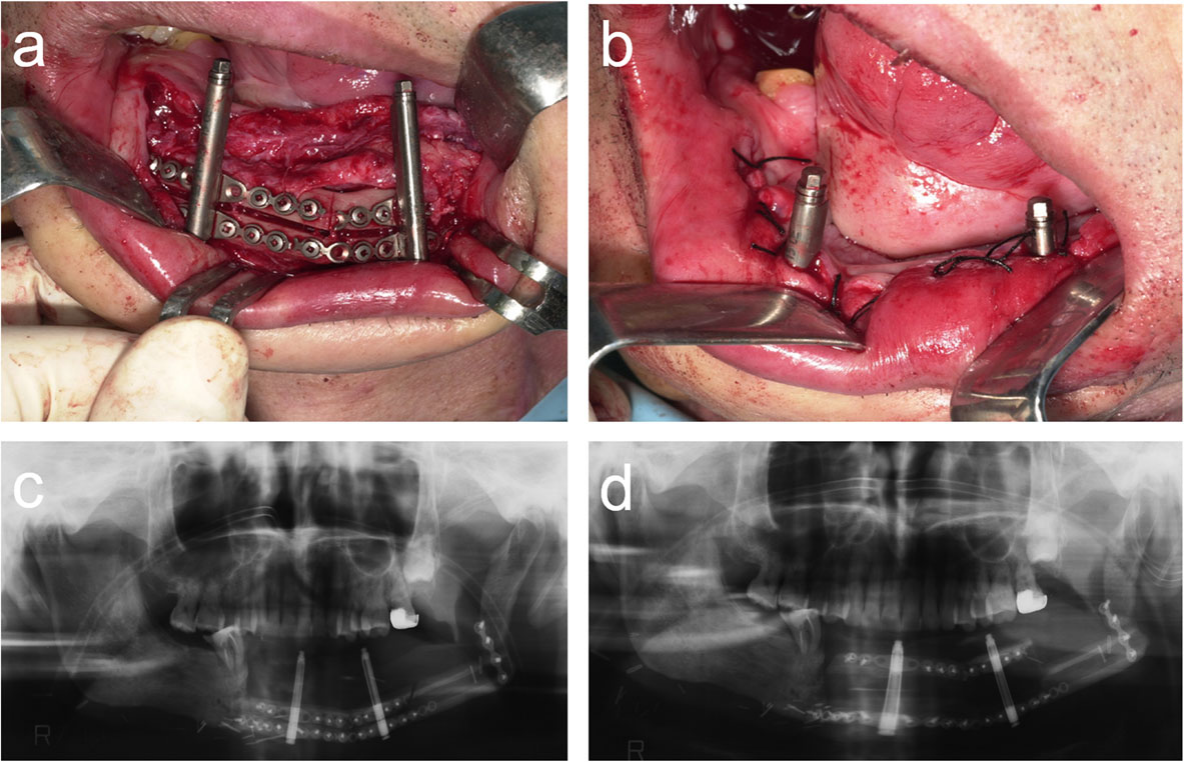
a, b Two distractors were fixed at the planned position. Orthopantomogram c 1 day postoperatively and d at the end of distraction osteogenesis
